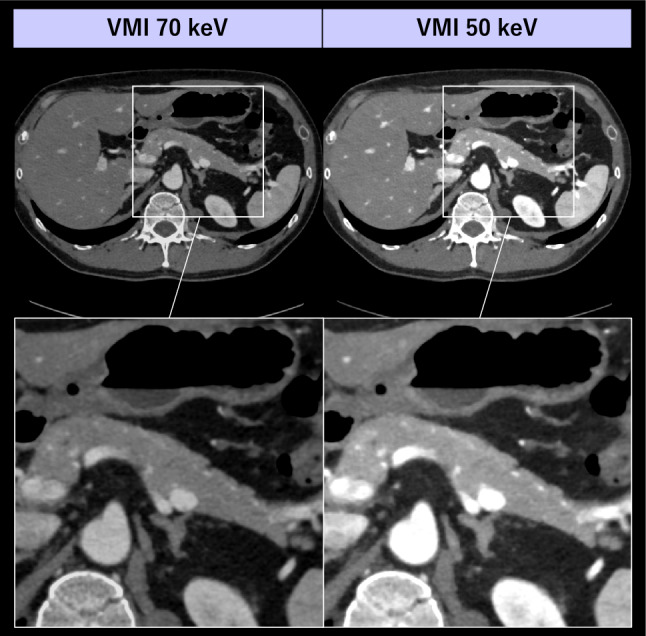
A 40-year-old man underwent abdominal CT with a low ICM dose protocol (350 mgI/kg) on a PCD-CT scanner. Compared with the VMI 70 keV images, iodine contrast of VMI 50 keV is considerably improved while preserving diagnostically acceptable image noise level. Image courtesy of Medical Scanning Tokyo
